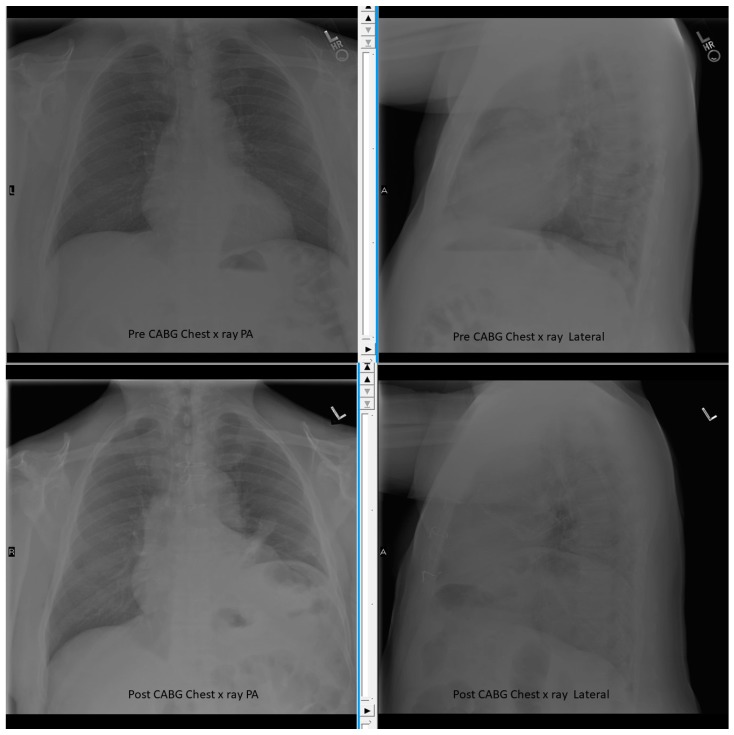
Chest X-ray Pre and Post Cardiac Bypass Surgery (CABG) showing elevated left diaphragm after surgery. The sternal metal suture wires also seen.
